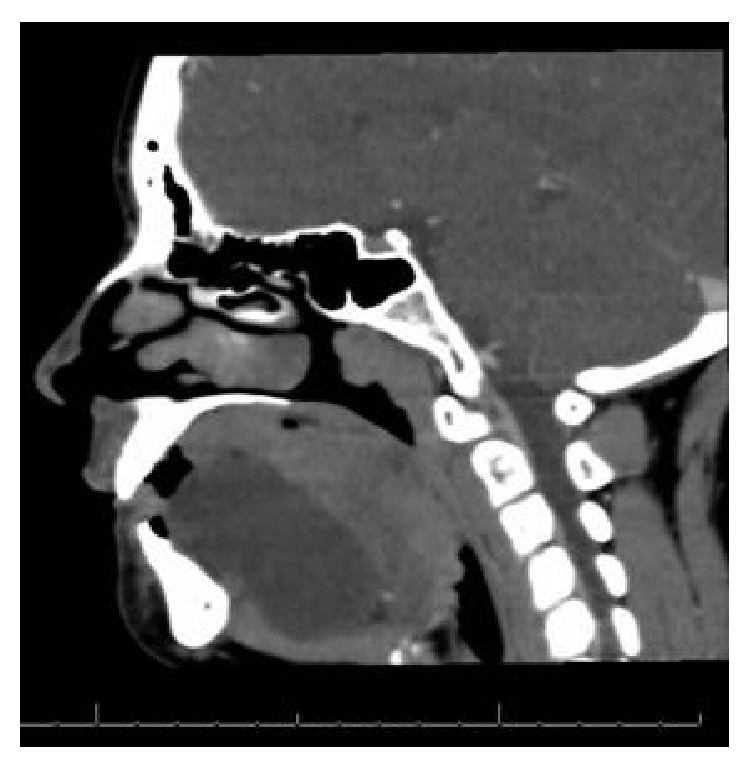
CT image demonstrating tracheal compression secondary to a large expanding ranula.
